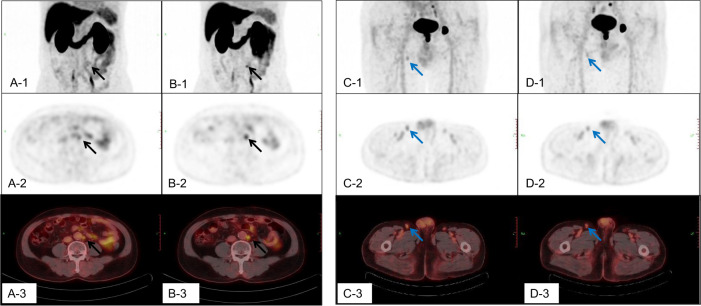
The ^18^F-DCFPyL uptake of malignant and benign lymph nodes in dual-phase PET/CT imaging. The A,B,C, D-1 were the maximum intensity projection (MIP) images; The A,B,C, D-2 were the cross sections of PET; and the A,B,C, D-3 were the cross sections of fusion images. The A, C-1, 2, 3 were for the early phase, and the B, D-1, 2, 3 were for delay phase. The A and B were the dual-phase ^18^F-DCFPyL PET/CT scan for a retroperitoneal lymph node metastasis. The short diameter of this lymph node was about 7 mm, SUVmax early was 3.0, SUVmax delay was 3.8, △SUVmax was 0.8, RI was 26.7%, SUV ratio early was 5.0, SUV ratio delay was 12.7, and ratio(SUV ratio delay/early) was 2.54. The C and D were the dual-phase ^18^F-DCFPyL PET/CT scan for a reactive hyperplastic lymph node. The short diameter of this lymph node was about 7 mm, SUVmax early was 2.1, SUVmax delay was 1.7, △SUVmax was -0.4, RI was -19.0%, SUV ratio early was 3.5, SUV ratio delay was 3.4, and ratio (SUV ratio delay/early) was 0.97.
